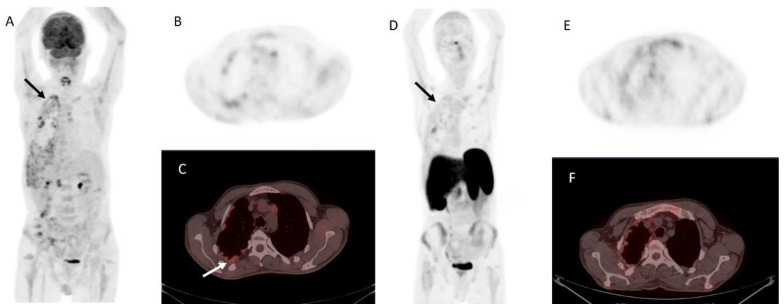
Metastatic parathyroid carcinoma on [^18^F]FDG PET/CT and [^68^Ga]Ga DOTA-TATE PET/CT. Legend: A 72-year-old patient with metastatic parathyroid carcinoma. He had elevated Ca levels of 13.5 mg/dL (8.8–10.6 mg/dL) and PTH levels of 11,663 (12–88 pg/mL) after repeated surgeries and tyrosine kinase inhibitor therapy. [^18^F]FDG PET/CT shows extensive pleural metastases ((A), whole-body MIP image, black arrow; (B), axial MIP; (C), PET/CT, white arrow). [^68^Ga]Ga DOTA-TATE PET/CT was acquired to evaluate options for peptide receptor radionuclide therapy ((D), whole-body MIP image; (E), axial MIP; (F), PET/CT). Unfortunately, there was a faint uptake lower than the physiological liver uptake, rendering the patient ineligible for PRRT.
